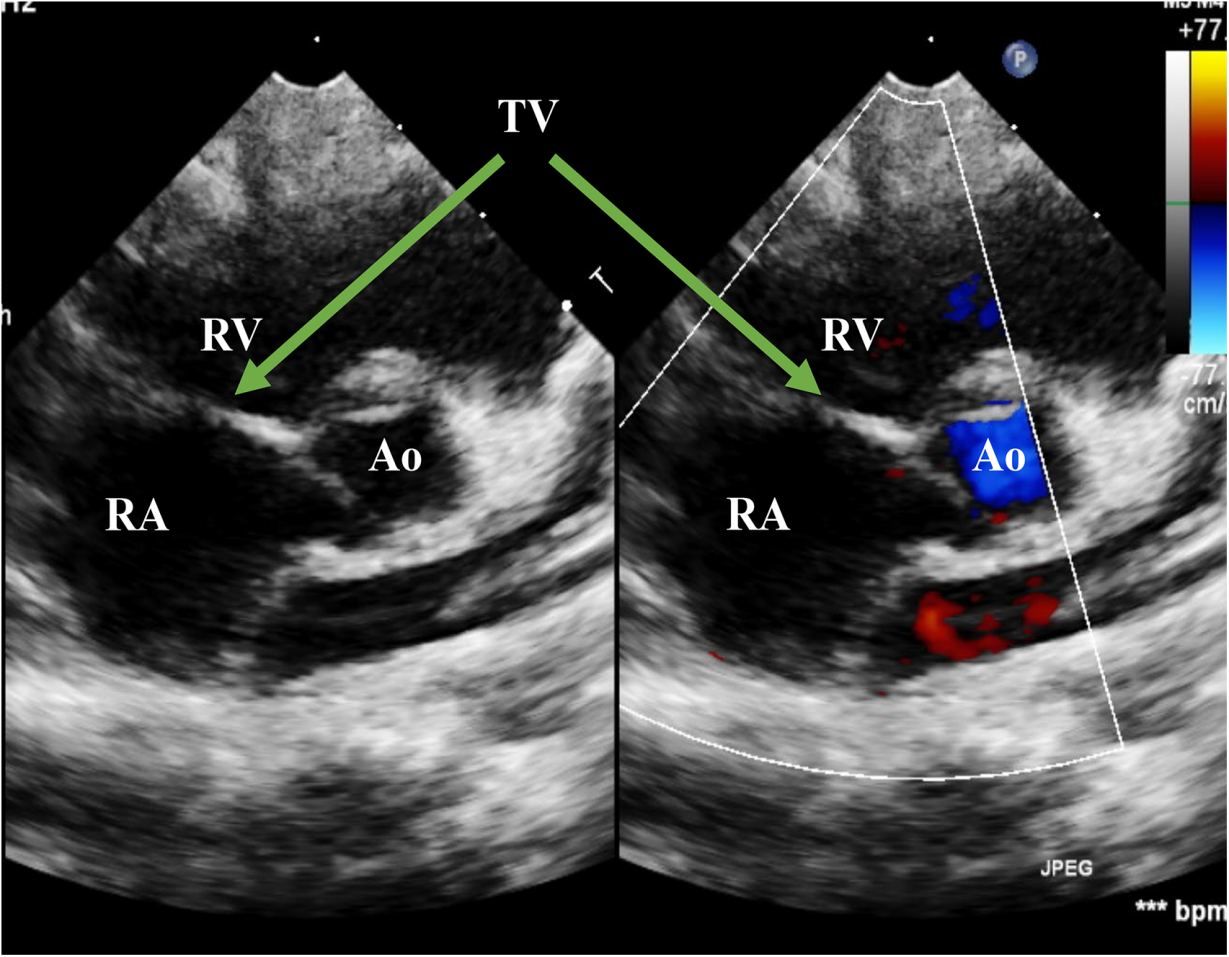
Echo at the latest visit in the PSAX view. The figure showed good coaptation of the TV in the systolic phase. PSAX, parasternal short-axis view; RA, right atrium; RV, right ventricle; Ao, Aorta; TV, tricuspid valve
